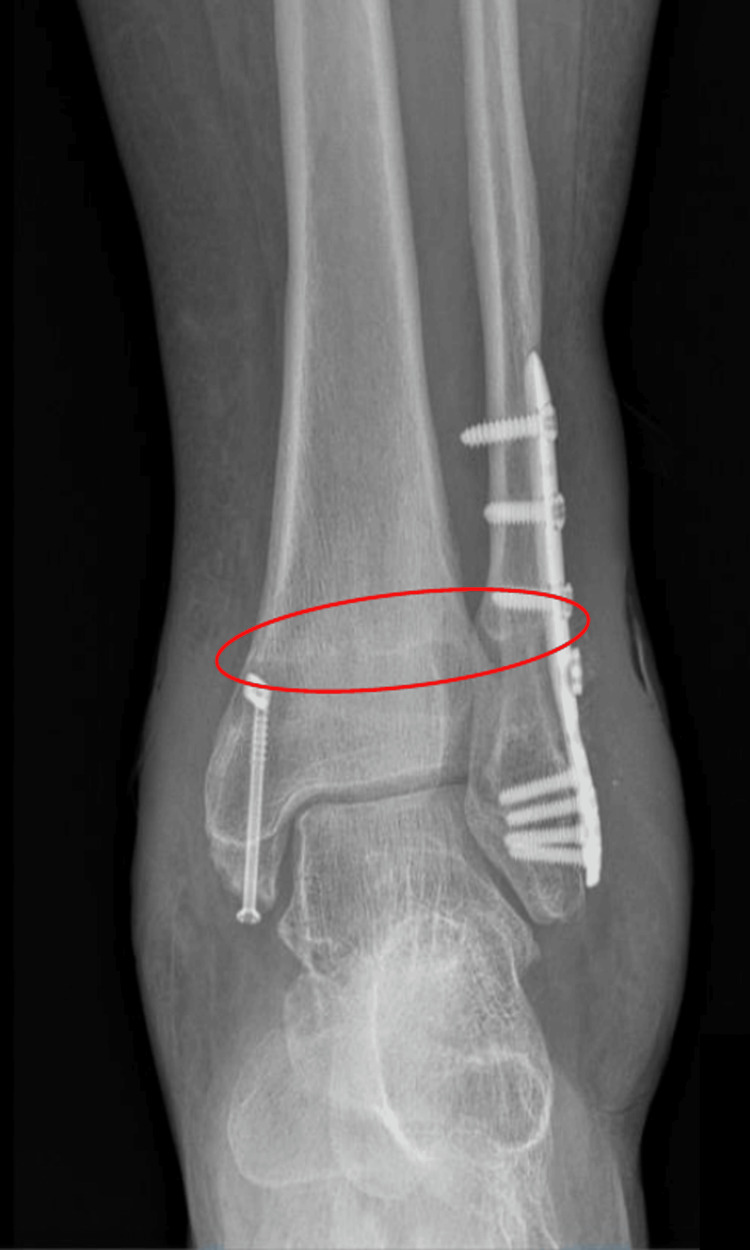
Anteroposterior X-ray of the ankle showing proper alignment with the presence of osteosynthesis material A radiolucent line corresponding to the course of the TightRope is visible (red circle).
